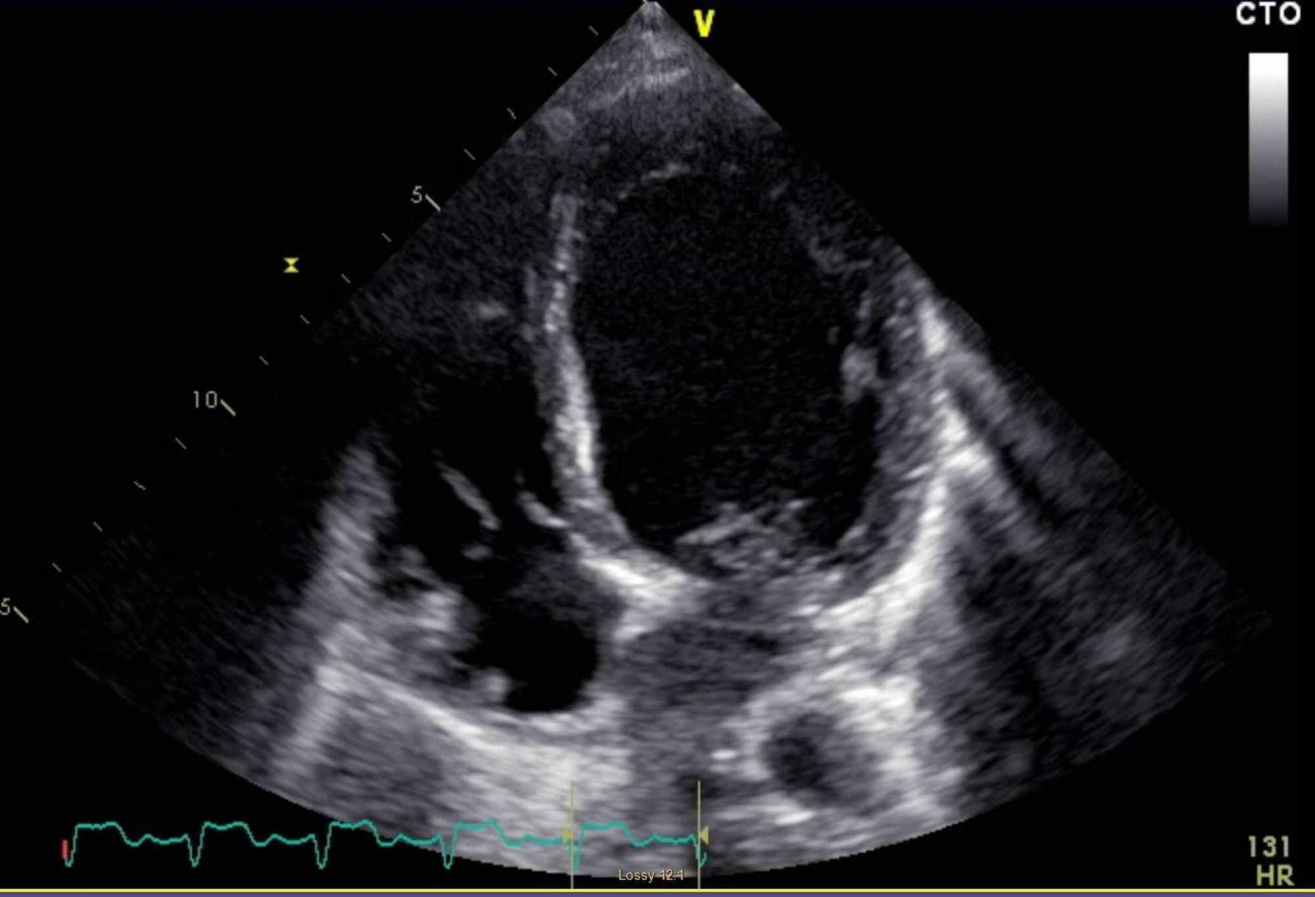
Transthoracic echocardiogram There is evidence of a severely dilated left ventricle with a measured ejection fraction of 15%.
